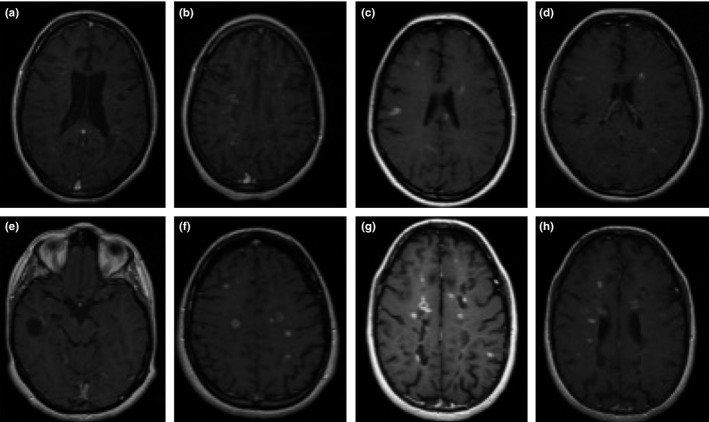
Axial T1 weighted magnetic resonance imaging (MRI) images after gadolinium (Gd) administration. The images demonstrated a high inflammation activity with elevated load of Gd‐enhancing lesions. (a) and (b) Patient 1; (c) and (d) patient 2; (e) and (f) patient 3; (g) and (h) patient 4
